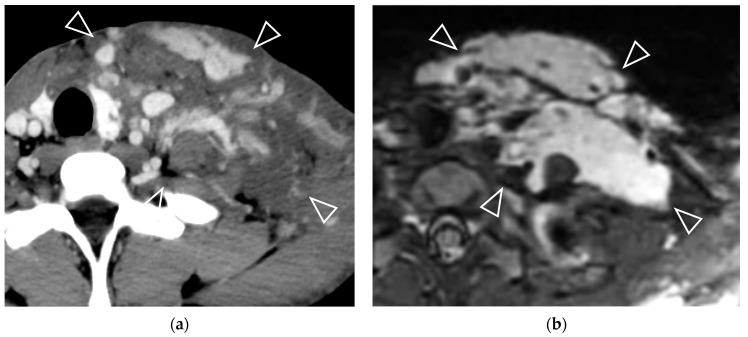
A 10-year-old boy with an infiltrative venous malformation on the left side of his neck. (a) A post-contrast CT neck showed an infiltrative hypodense lesion with incomplete contrast enhancement (arrowheads). (b) Axial T2-weighted with fat suppression image showed a multiloculated cystic lesion with high signal intensity involving multiple compartments of the left-sided neck (arrowheads). Following the third session of bleomycin injection, the patient observed an improvement in symptoms, accompanied by a reduction in size of over 50%.
